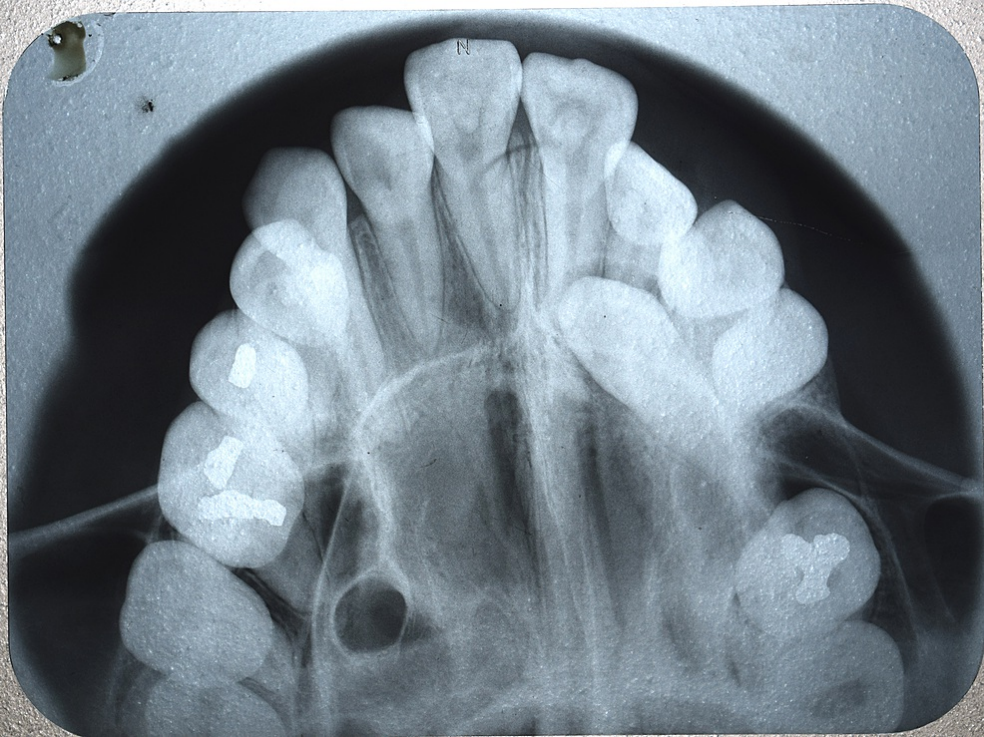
Occlusal radiograph with palatally impacted 23
